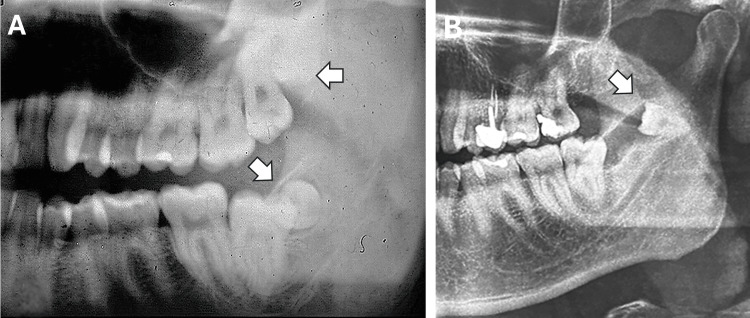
Conventional panoramic radiograph views. (A) Previous panoramic radiograph taken 10 years earlier showing two impacted rudimentary fourth molars (upper and lower) on the left side (arrows). (B) Current panoramic radiograph showing the ectopically positioned, eumorphic mandibular fourth molar in the sigmoid notch (arrow). An ascending radiolucent image was seen extending from the impacted third molar to the sigmoid notch.
